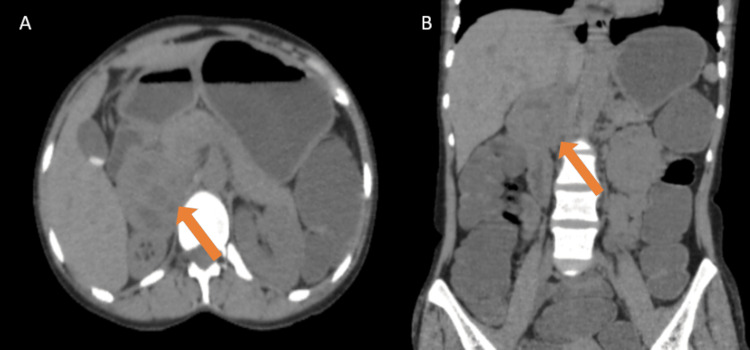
Plain axial (A) and coronal (B) CT images show an iso-hypodense lesion in the right adrenal region having smooth margins (orange arrows). CT: computed tomography
